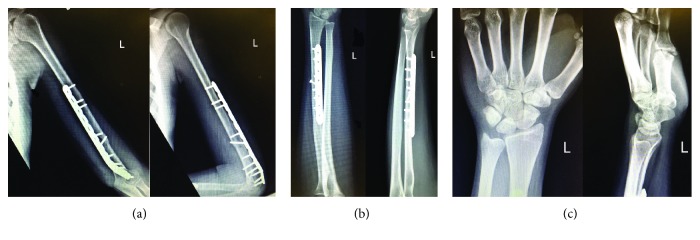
One year postoperative AP and lateral radiographs of the left humerus (a), forearm (b), and wrist (c) demonstrating healing of both humeral and radial shaft fractures and maintenance of reduction of the distal radioulnar joint.
